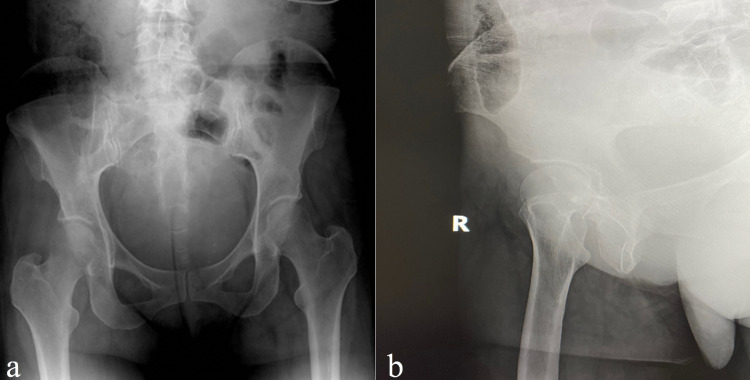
Radiographs of the right hip and pelvis postreduction. (a) Anteroposterior (AP) view of the right pelvis and (b) lateral view of the right hip. These images show a well-reduced right hip joint with the femoral head and acetabulum in appropriate alignment.
